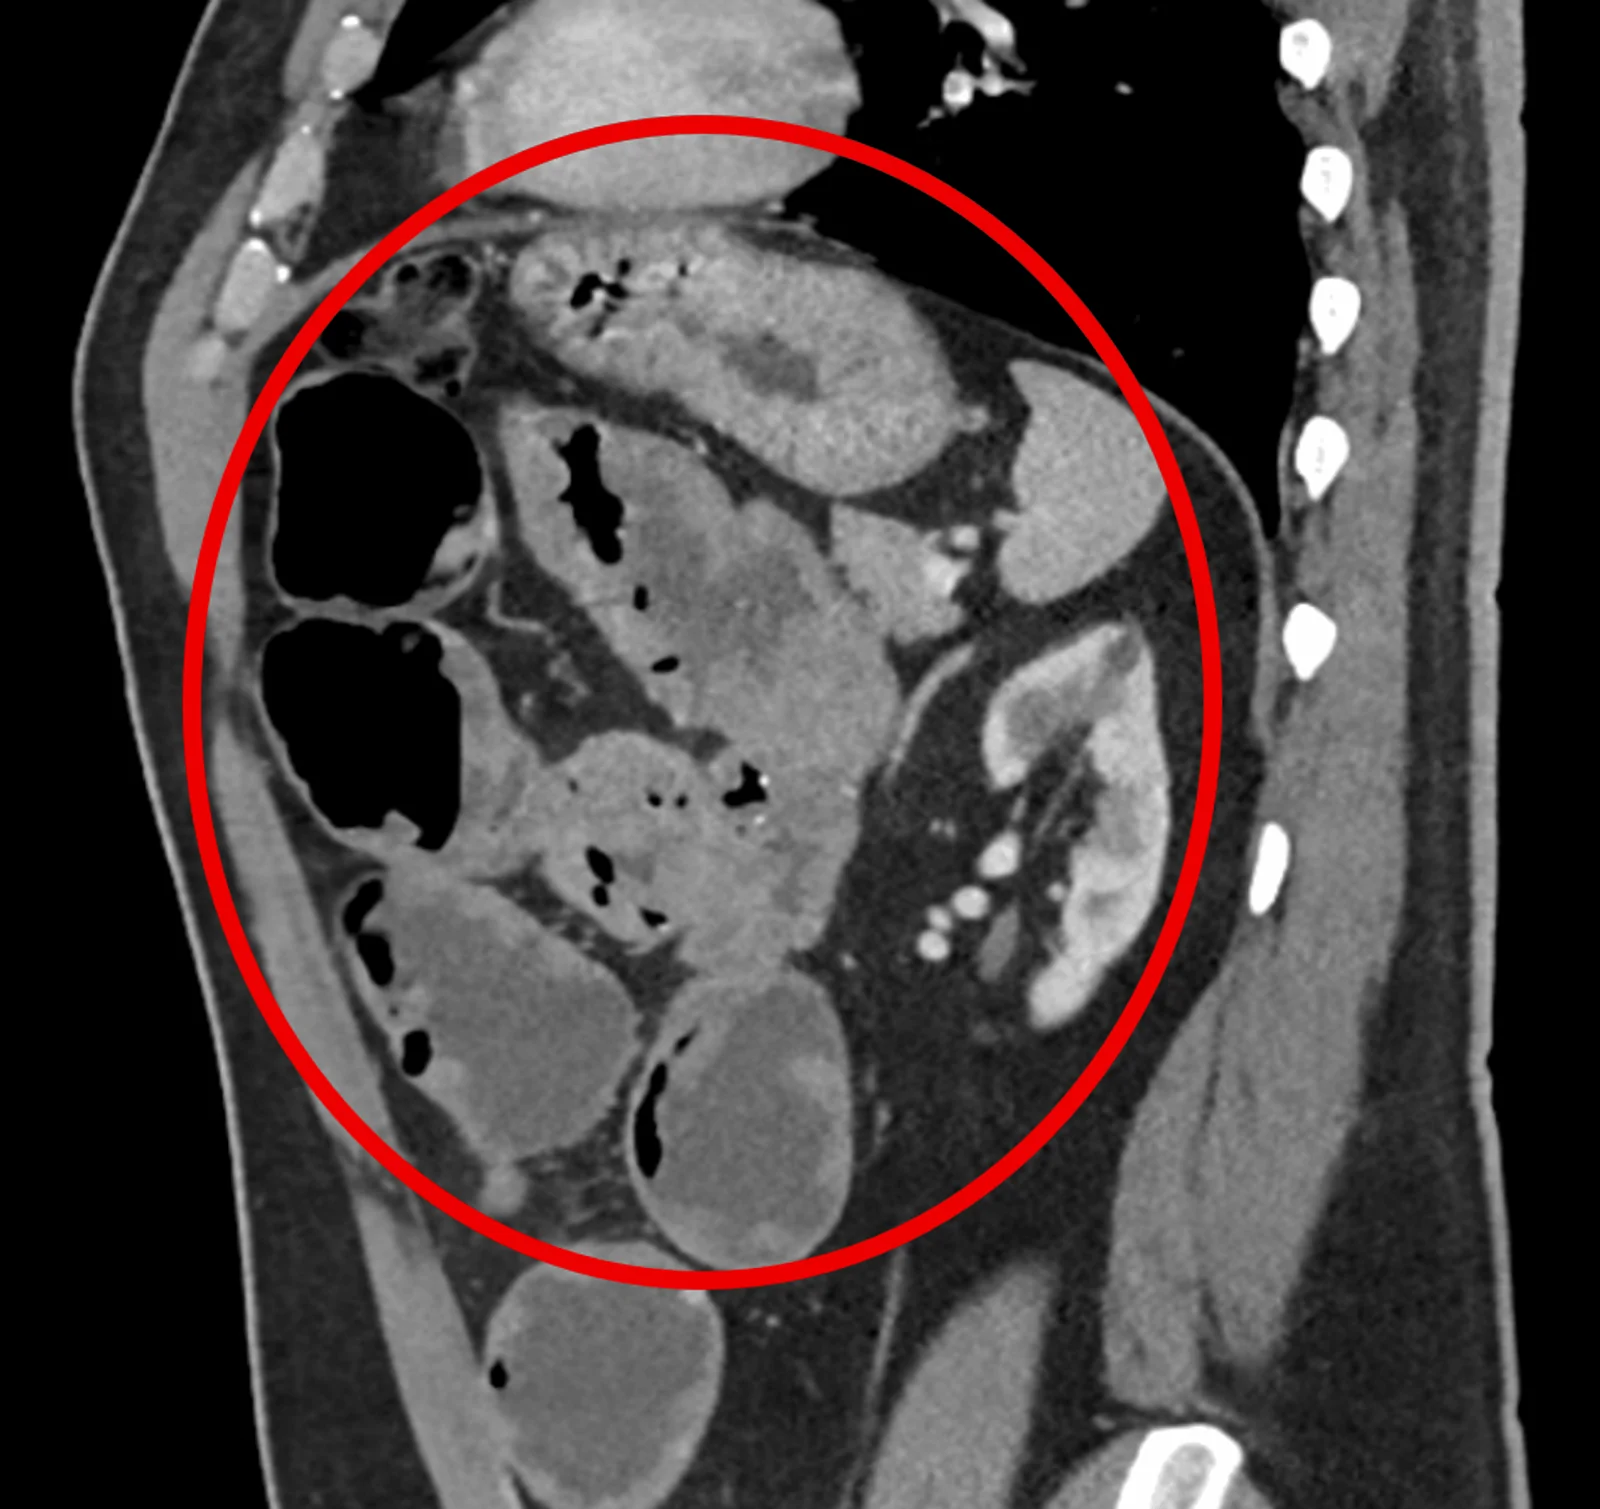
Sagittal CT abdomen without contrast showing segments of small bowel with thickened bowel walls.
